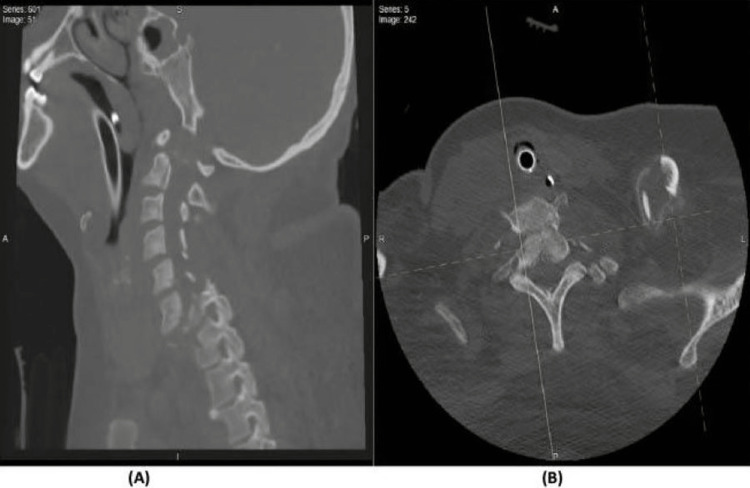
CT scan of C5-6 fracture involvement (A) Cervical spine CT scan sagittal cuts demonstrating a comminuted fracture of C5 transverse process, bilateral pedicles, and spinous process; (B) cervical spine CT scan axial cuts demonstrating the corresponding levels of C5 and C6–C7.
